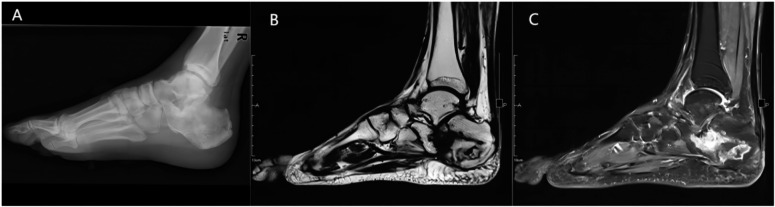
Imaging manifestations of diffuse osteomyelitis. A. X-ray showed an irregular arrangement of bone trabeculae and heterogeneous bone density. B and C. MRI showed a bone fracture in the heel bone, a lamellar low signal shadow in the heel bone on the T1-weighted image, and an irregular large lamellar high signal shadow in the heel bone on the T2 compression lipid image
